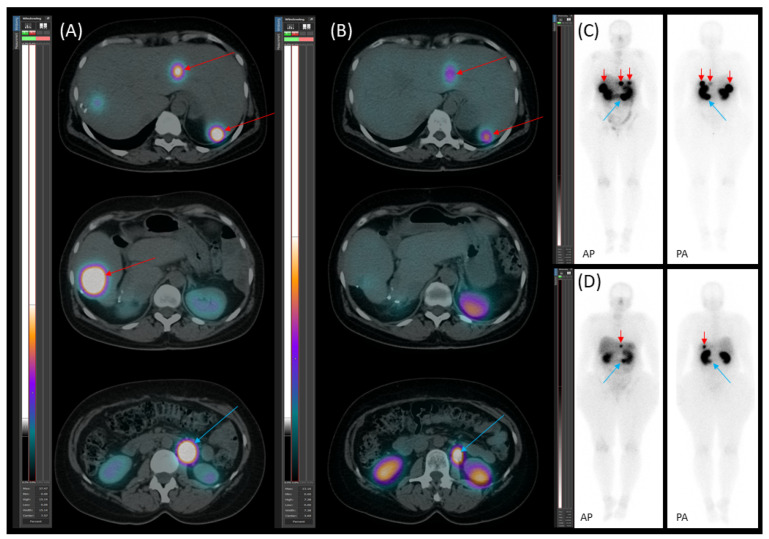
[^177^Lu]Lu-DOTA-TATE therapy response. Transaxial (A,B) SPECT/CT images and planar images (C,D) (AP—anteroposterior; PA—posteroanterior) obtained after the first (A,C) and fourth (B,D) therapy cycles with [^177^Lu]Lu-DOTA-TATE. The images demonstrate a reduction in both the number and activity of somatostatin receptor (SSTR)-positive lesions in the liver (red arrows), as well as a decrease in the size and activity of a para-aortic lymph node metastasis (blue arrow) following therapy.
